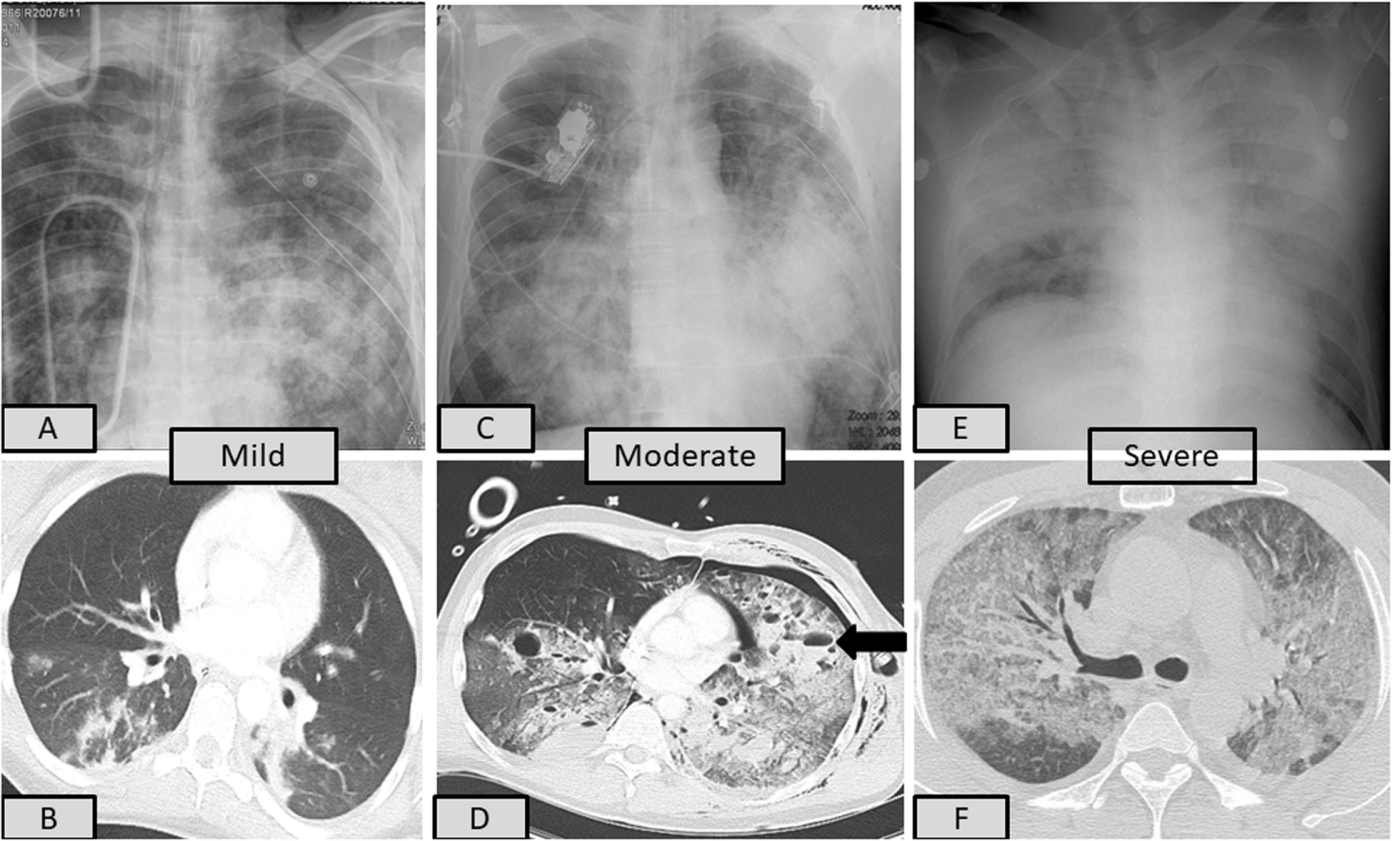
Distribution of pulmonary contusion on X-ray and the corresponding CT-scan. a Chest X-ray showing mild contusion. b CT scan showing mild contusion (mainly on the right side). c Chest X-ray showing moderate contusion (mainly on the left side). d CT scan showing moderate contusion with the presence of multiple pneumatoceles (arrow). e Chest X-ray showing severe contusions on both sides. f CT scan showing severe contusion on both sides
